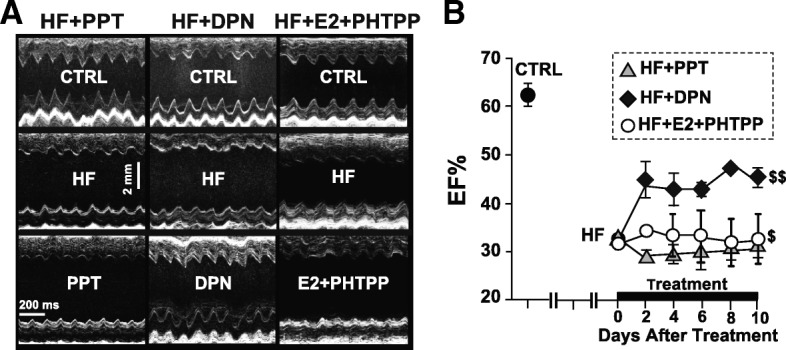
ERβ-agonist, but not ERα-agonist, significantly improves HF. a Examples of M-mode images of the parasternal short axis view by echocardiography before surgery (CTRL), in heart failure (HF) and 10 days after treatment with an ERα-agonist (PPT, left panels), ERβ-agonist (DPN, center panels), or E2 together with the ERβ-specific antagonist PHTPP (right panels). b Averaged EF as a function of time in PPT (triangles, *n* = 6), DPN (diamonds, *n* = 7), and E2+PHTPP (circles, *n* = 4). ^$^*P* < 0.05 vs. PPT and ^$$^*P* < 0.001 vs. PPT
